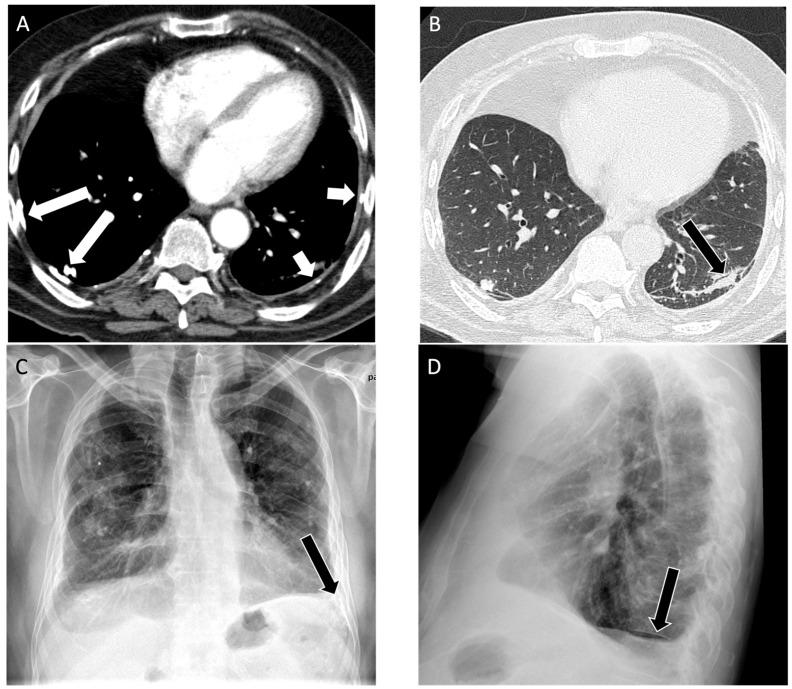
A 73-year-old male with a history of remote 16 pack year smoking history and asbestos exposure as a retired plumber working on pipe and broiler insulation, diagnosed with asbestos-related pleural disease. (A) Axial computed tomography shows asbestos plaques on the right (long arrows) and diffuse pleural thickening on the left (short arrows) on the soft tissue window; (B) on the lung window, a subpleural band opacity is seen in the left lower lobe (black arrow); (C) posteroanterior view and (D) lateral view chest radiographs show diffuse pleural thickening with calcification, best appreciated by costophrenic angle blunting (black arrows).
